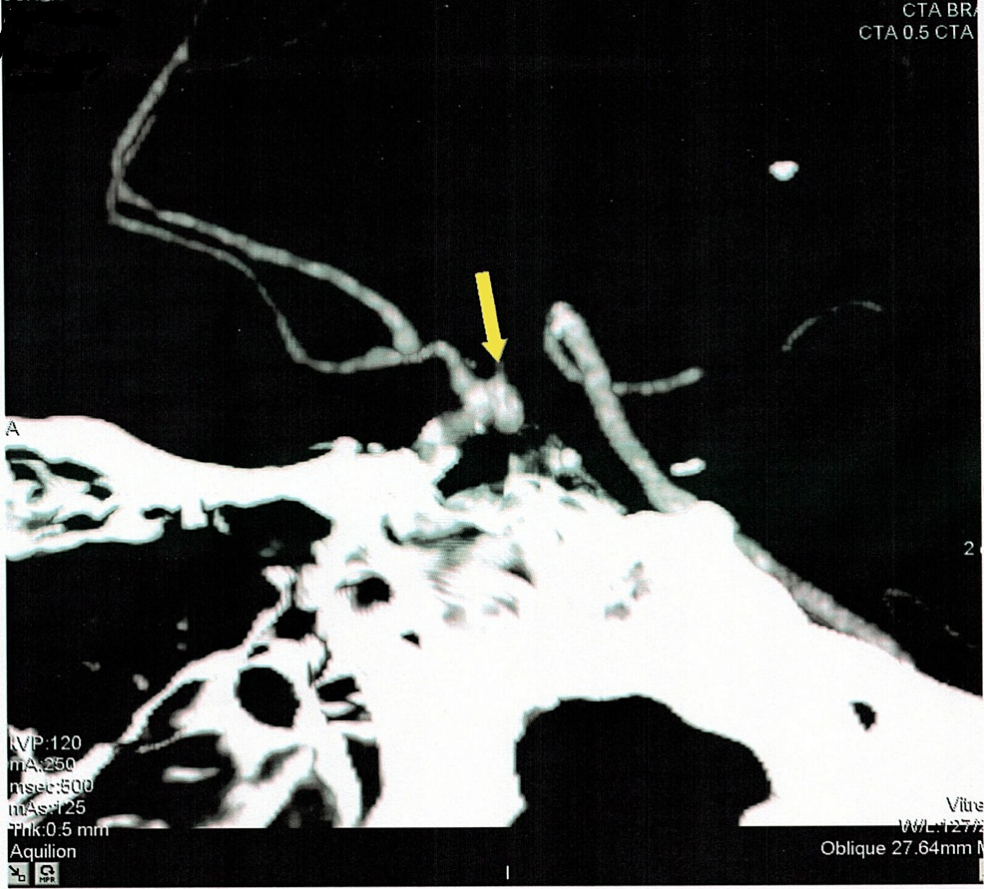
Computed tomography angiogram depicting the location of the aneurysm
